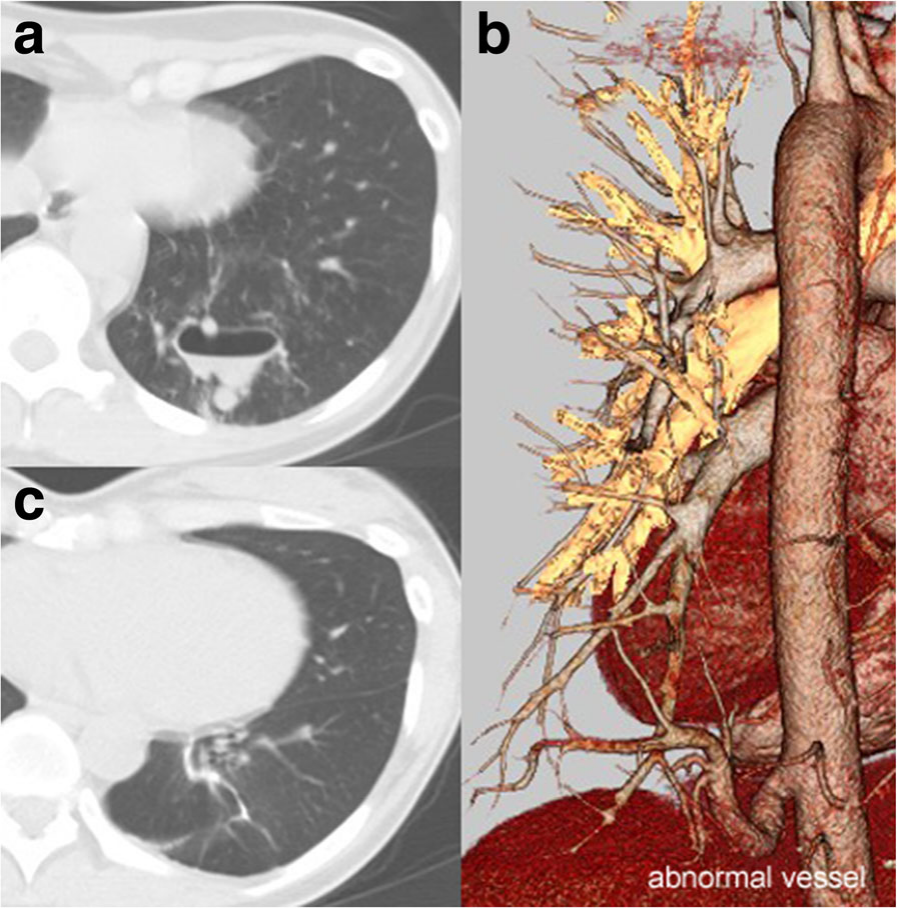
CT images obtained before and after treatment. a Chest CT scan showing several cystic lesions with niveau formation and consolidation in left S10 without communication with the bronchus. b Three-dimensional CT scan showing an abnormal artery branching from the descending aorta and normal pulmonary vein. c Chest CT scan obtained at 2 years after surgery showing good expansion of residual left lower lobe with no consolidation
